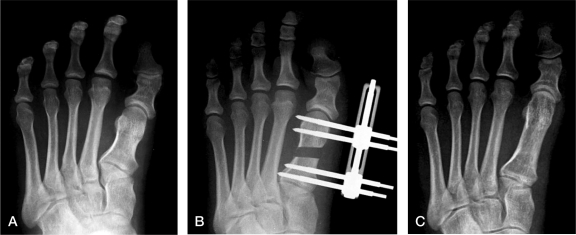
A. Preoperatively: shortening of the first metatarsal. B. Patient undergoing lengthening of the first metatarsal by distraction osteogenesis. C. Excellent final radiographic result.
